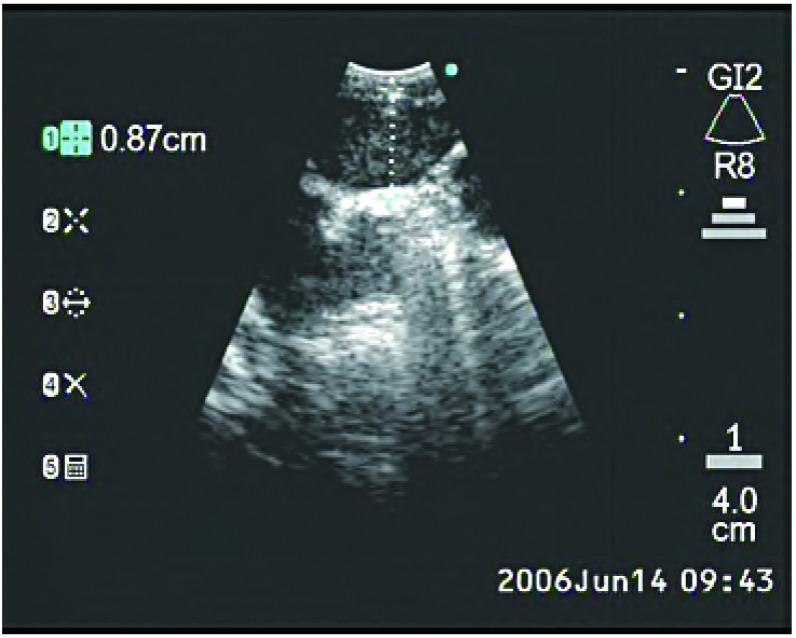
支气管内超声所见左侧气管旁淋巴结 Endobronchial ultrasound of left paratracheal node
